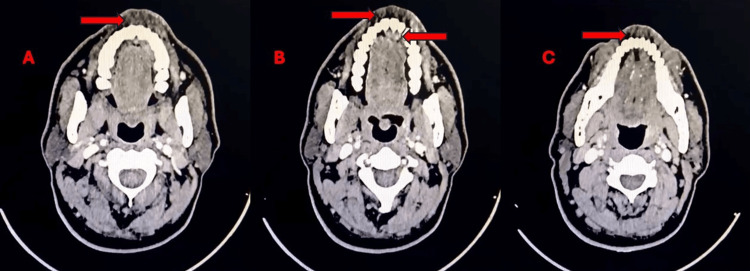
(A-C) Contrast-enhanced computed tomography (CECT) scan of head and neck (axial view) showing enhancing mucosal thickening in the left upper gingival region (red arrows)
